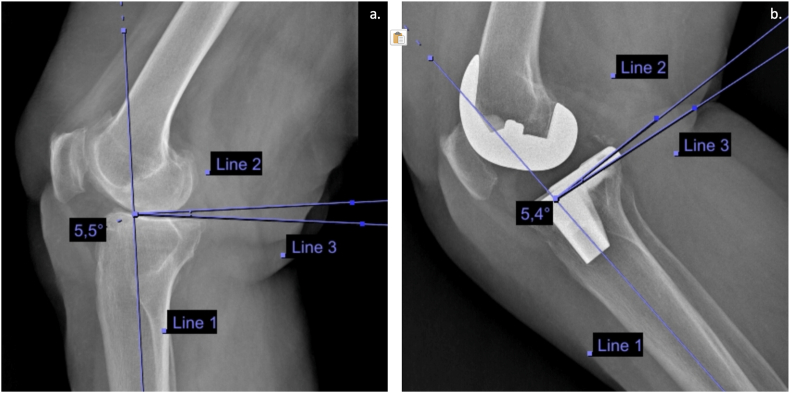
(a) Preoperative lateral X-ray of a left knee. The preoperative PTS was calculated with the tibial proximal anatomic axis method and its value was 5.5°. (b) Postoperative lateral X-ray of the same left knee in which the PTS was maintained. PTS: Posterior tibial slope; °: degree.
